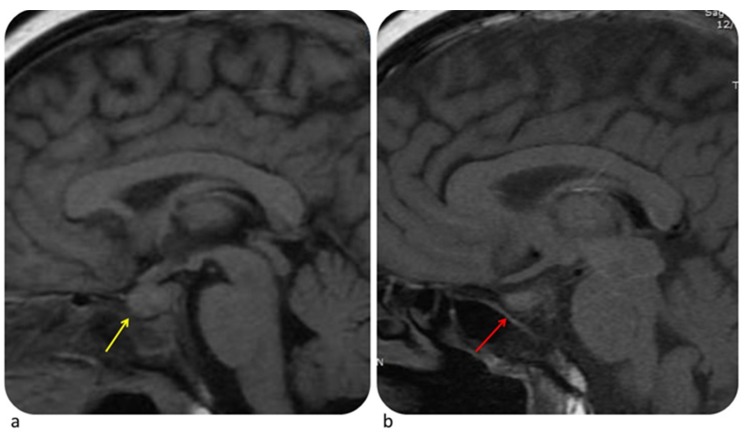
Hypophysitis in a 54-year-old woman with metastatic melanoma treated with ipilimumab. MRI performed at time of headache shows homogeneously enlarged pituitary gland (a, yellow arrow). Ipilimumab was stopped and patient was given hormone replacement therapy. MRI obtained one month later shows decrease in size of pituitary gland (b, red arrow). © Department of Neuroradiology, University of Pisa.
